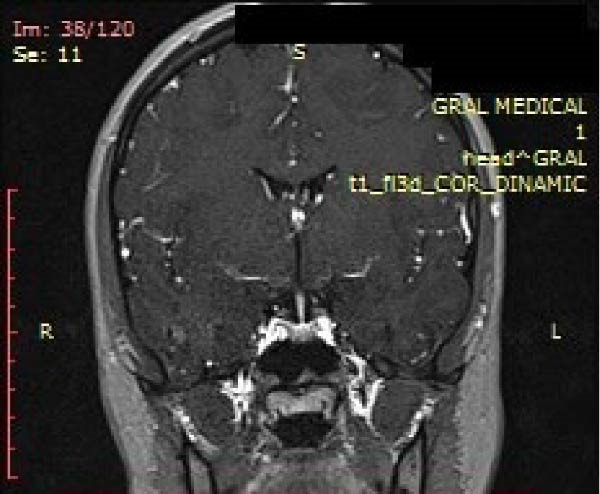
Coronal T1‐weighted postcontrast dynamic MRI showing asymmetric contrast enhancement in the pituitary gland. A small hypointense area on the left side demonstrates delayed enhancement.
